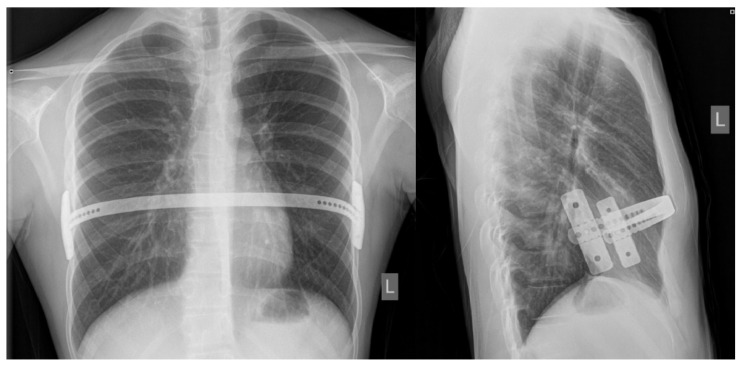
Bar position at postoperative chest X-ray: posteroanterior (left) and laterolateral (right) views.
